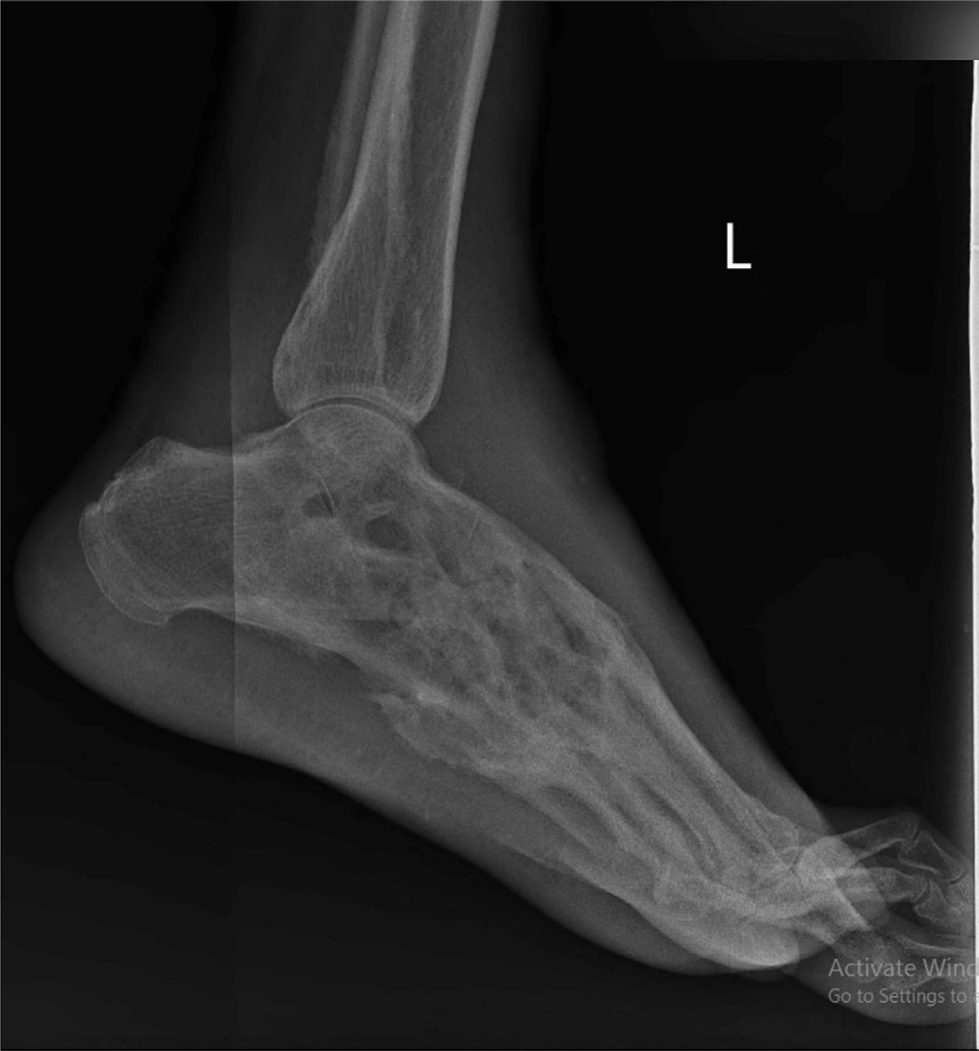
Radiograph foot (Lateral projection) showing ill-defined lytic and sclerotic lesions in tarsal bones with ankylosis at inter-tarsal and metatarsophalangeal joints.
